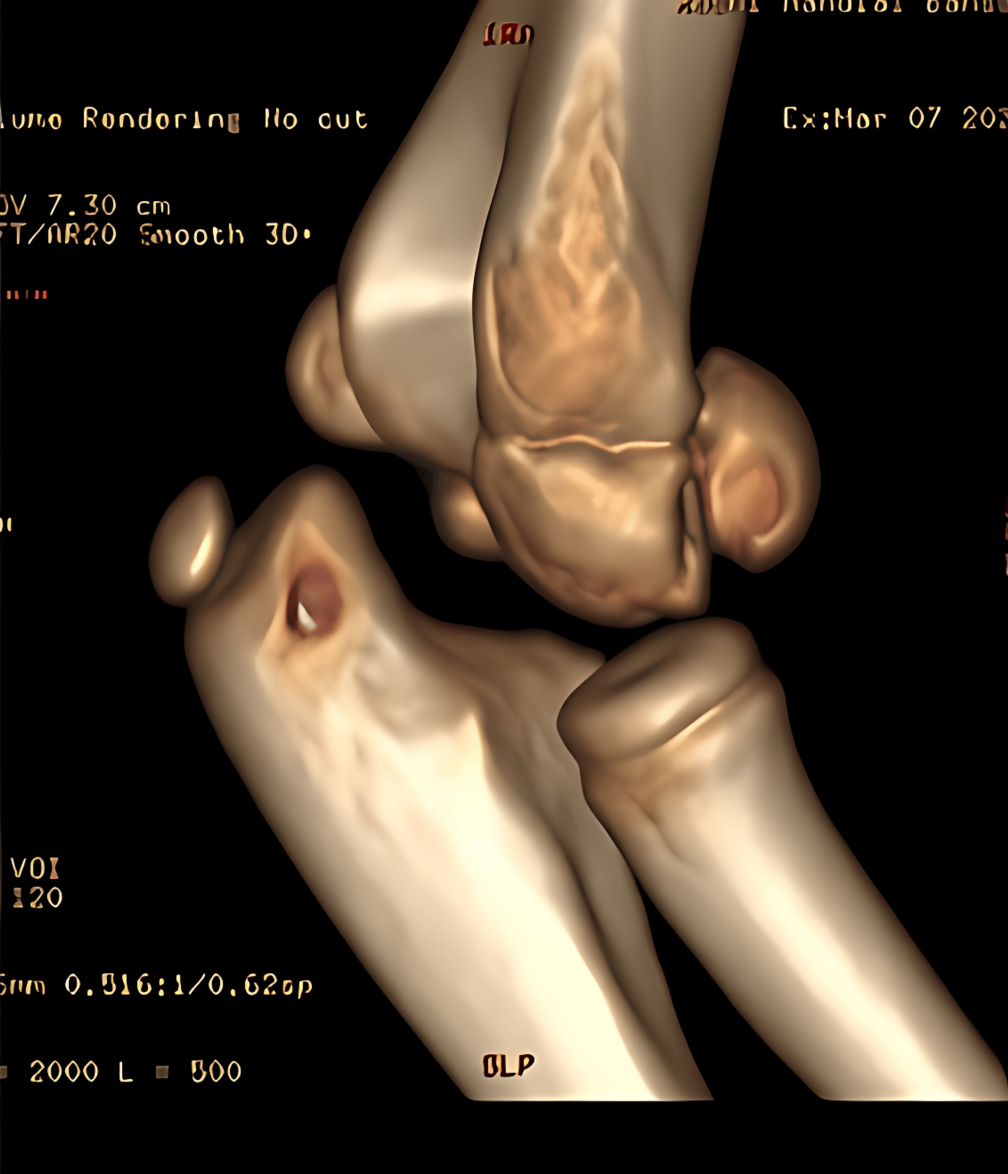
Preoperative CT scan of case 3 showing capitellum fracture
